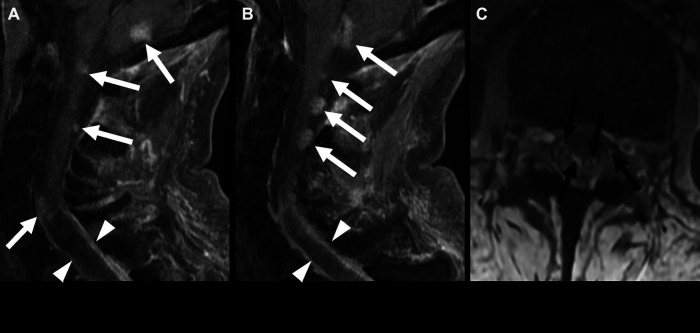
Spinal ependymoma, MYCN amplified, in a 46-year-old woman. Sagittal post-contrast MRI (A,B) shows multiple enhancing nodules in the visualized posterior fossa and cervical spinal cord (white arrows). A partially cystic lesion extends from the mid-cervical spine into the thoracic spine (arrowheads). Multiple enhancing nodules in the cauda equina are consistent with leptomeningeal spread (C) (black arrows).
